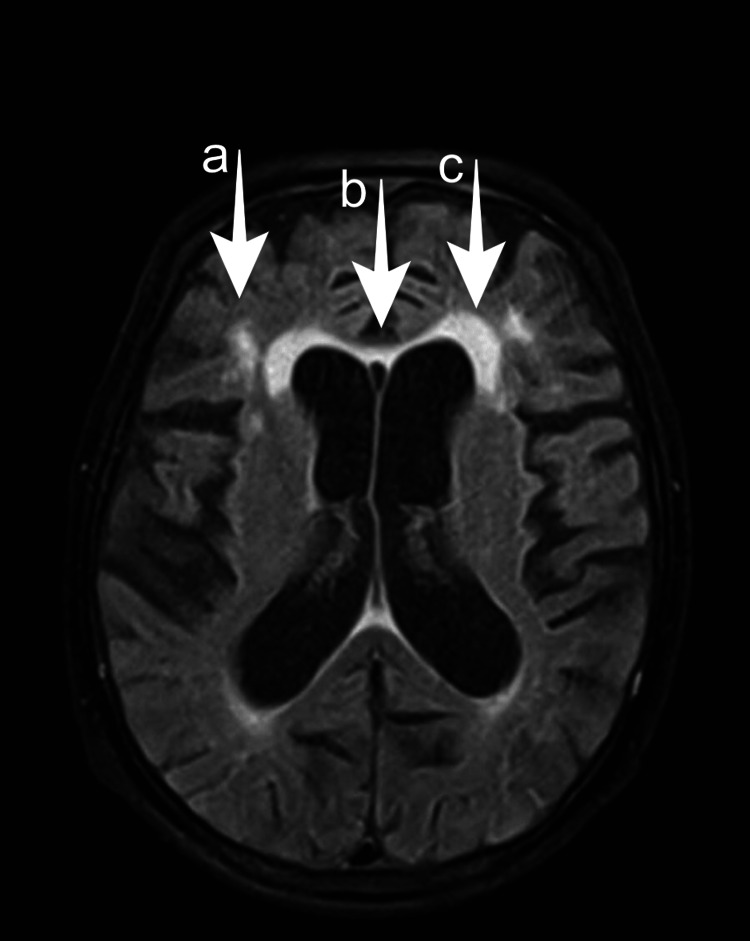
MRI axial T2 FLAIR images showing hyperintense lesions in (a) deep periventricular matter, (b) corpus callosum, and (c) deep white matter. FLAIR: fluid-attenuated inversion recovery
